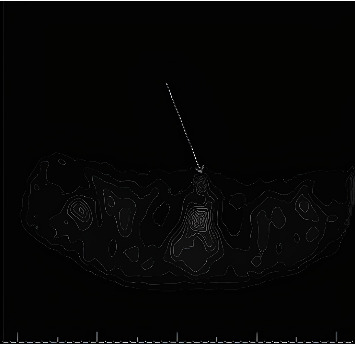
SPECT scan of parathyroid showing a focus of activity at the left thoracic inlet.
